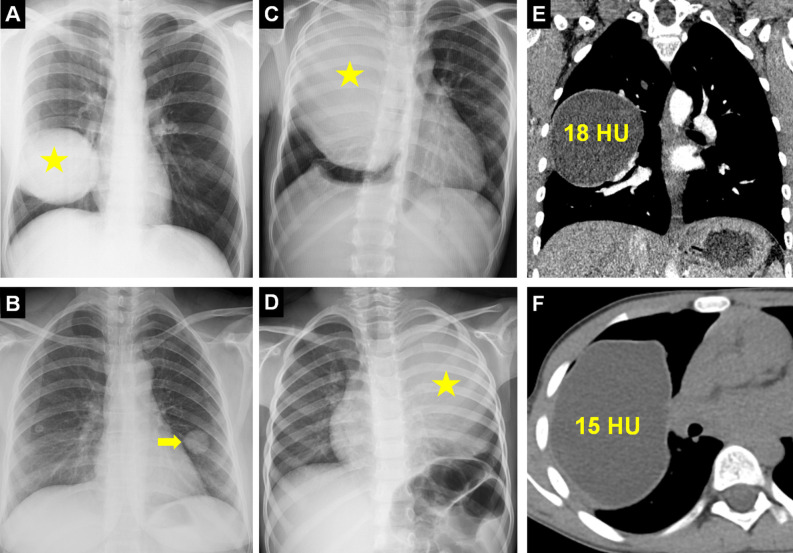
Radiological presentation of intact pulmonary hydatid cysts (HCs). Chest radiograph (A) shows a well-circumscribed, round, and homogeneous opacity (asterisk) due to an intact HC in the right lung. (B) Presentation of an early-detected pulmonary HC as a solitary pulmonary nodule (arrow). (C) Giant HC cases presenting with mediastinal shift in the right lung and (D) in the left lung (asterisks). Confirmation of the cystic content of the lesions via density measurements (Hounsfield Unit - HU) taken on coronal (E) and axial (F) computed tomography images.
